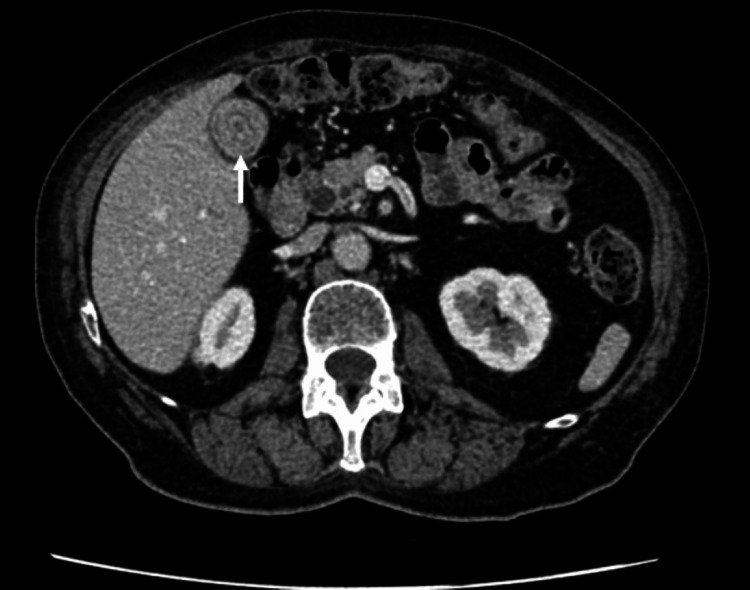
Axial portal venous CT shows a gallstone within the gallbladder (white arrow) with no acute changes.
